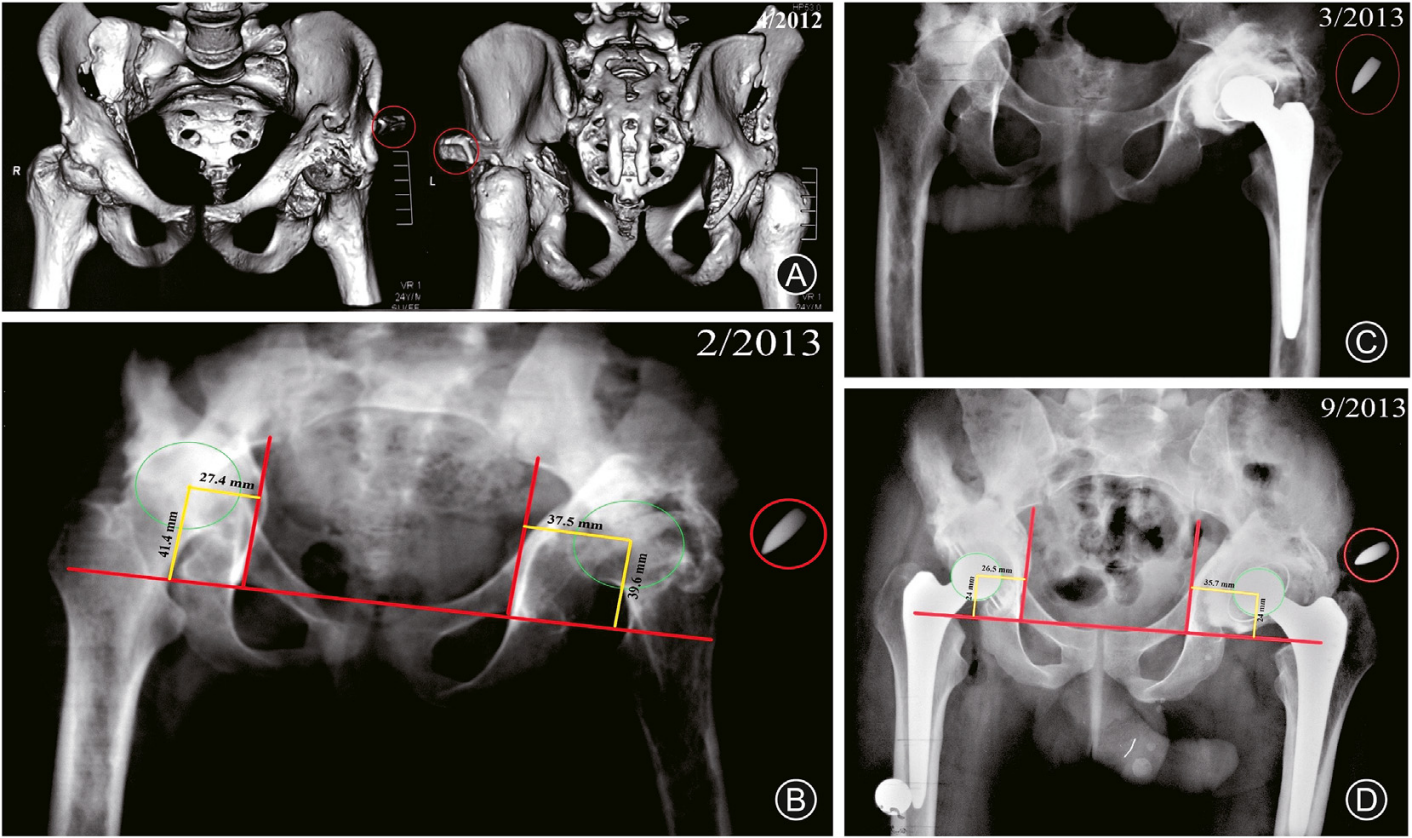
(A) Pelvis CT at the time of the patient's first presentation at the trauma unit showing bilateral iliac wing and acetabular fractures and a retained bullet (red circle). (B) Preoperative plain radiographs showing bilateral hip arthritis, proximal migration of the femoral head bilaterally and type ⅢA acetabular bone defect according to Paprosky classification. (C)& (D) Postoperative radiographs showing reconstruction of the hips bilaterally by impaction bone grafting and reversed hybrid total hip arthroplasty.
